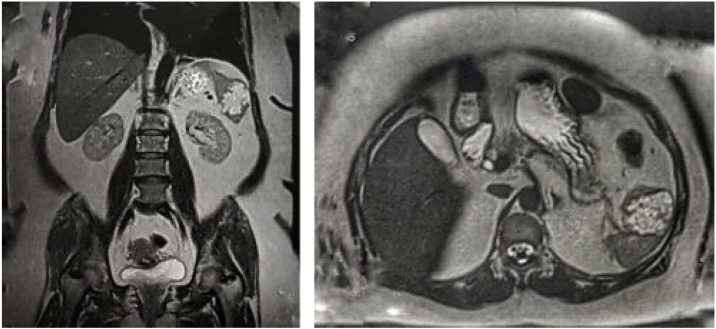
Noted splenic mass, approximately 5 cm in the patient’s MRI which appeared hyperintense in T1-weighted images, implying the fluid contents of the mass.
